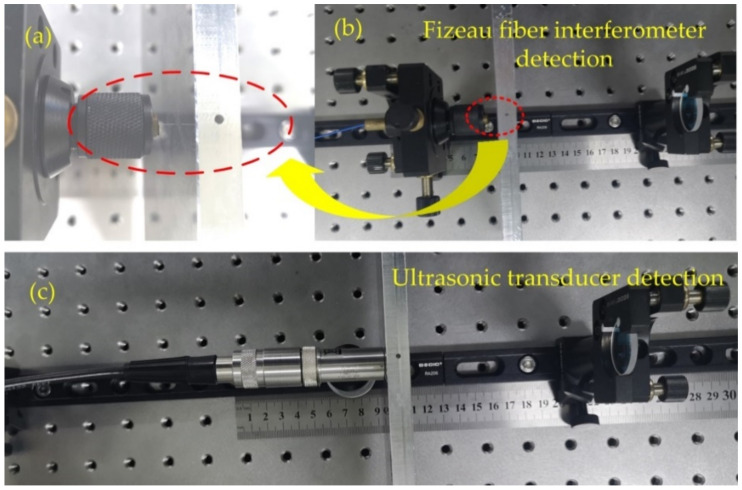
Fiber end face of the Fizeau fiber interferometer (a), and the interferometer (b) and ultrasonic transducer (c) detection.
